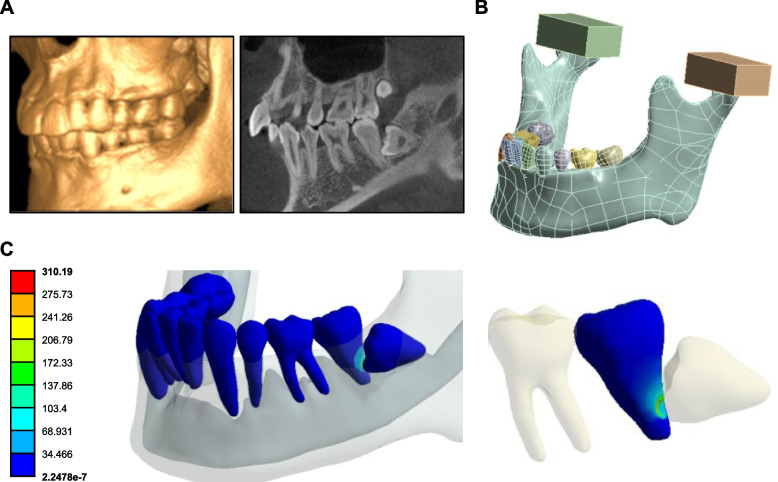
The ERR of M2M was caused by the force that EIM3M exerted on the distal root of M2M. A The cone-beam tomography images of one selected participant were displayed by i-CATVision. B The three-dimensional (3D) reconstruction model was created from the cone-beam tomography data. C Equivalent (von Mises) stress, unit: MPa. The area of high energy dissipation was observed in the contact region between the EIM3M and the M2M
